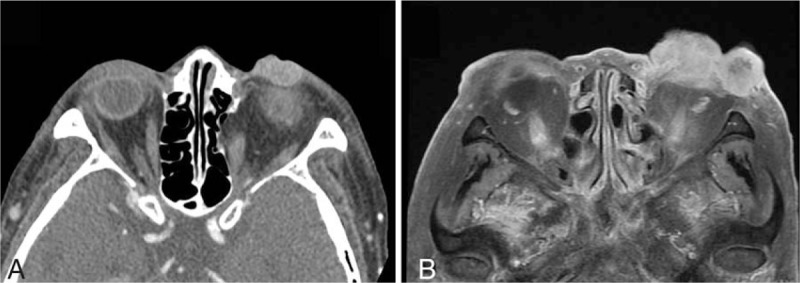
Computed tomography image of the left lower eyelid lesion at the initial visit (A), and a magnetic resonance image 4 weeks after the initial visit (B).
